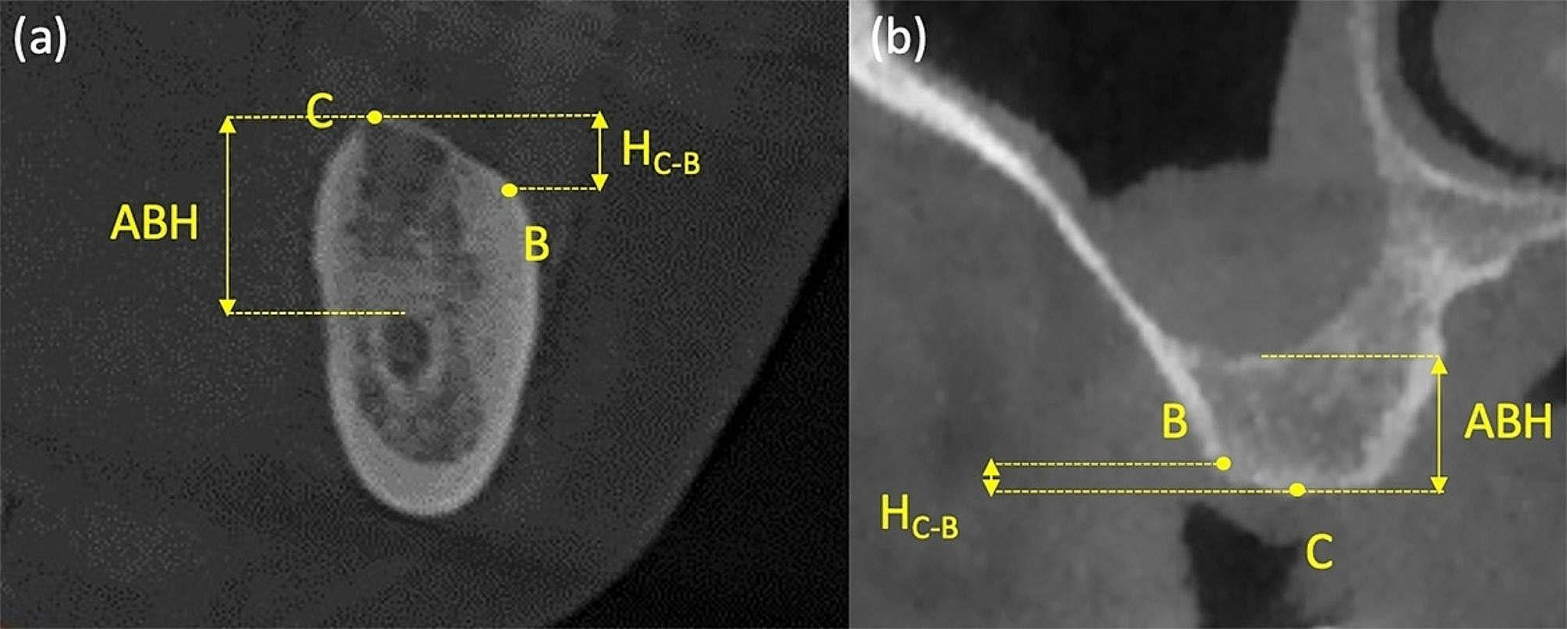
Radiographic measurements on CBCT images. Slices of (a) mandibular and (b) maxillary edentulous molar sites. C: alveolar crest; B: most coronal point of the buccal bone plate; H_C−B_: the height discrepancy between the alveolar crest and the buccal bone plate; ABH: alveolar bone height
